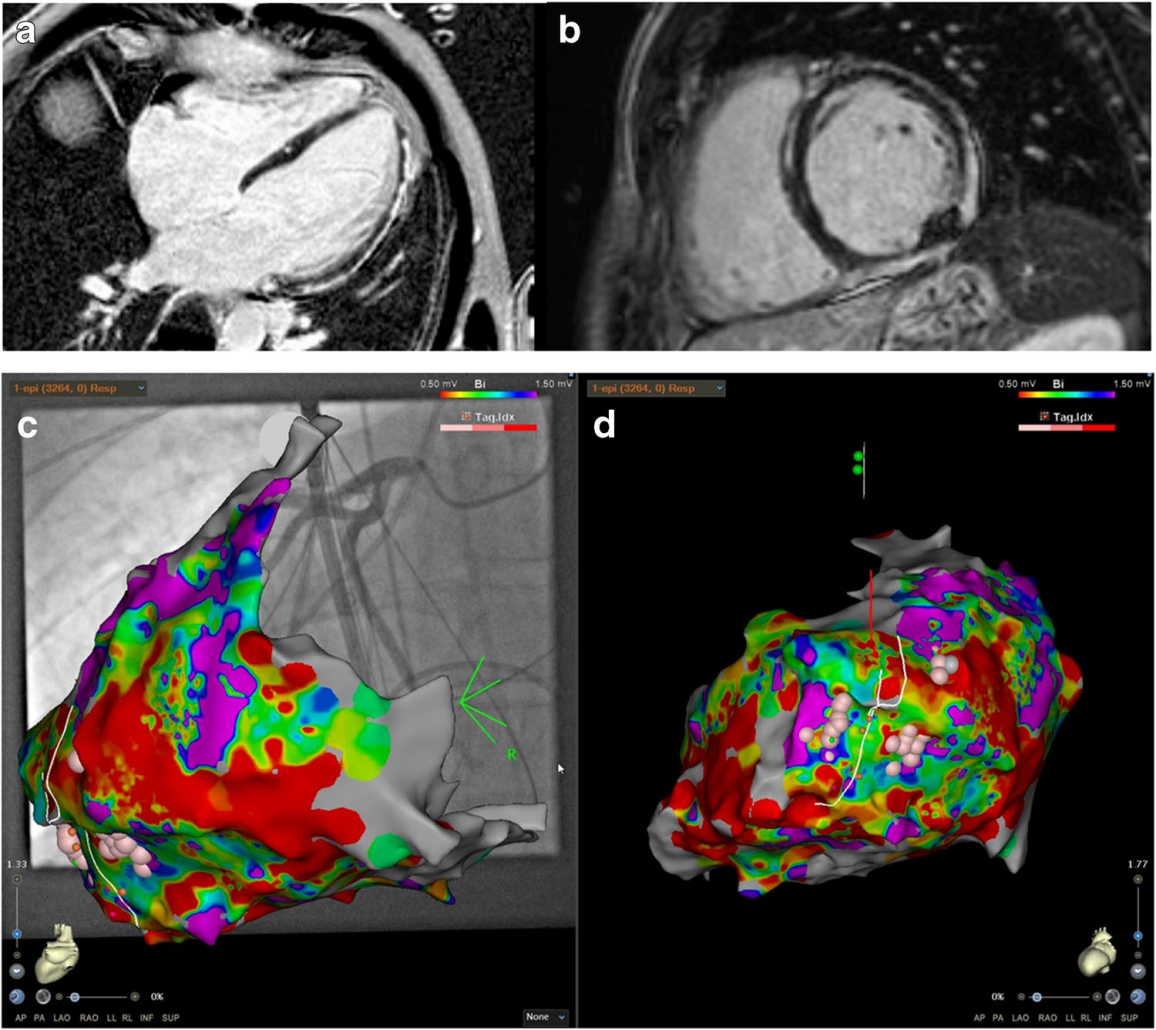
Preprocedural imaging using LGE-CMR to identify epicardial/midmyocardial substrate (A + B). Correspondingly, epicardial (C + D) map. Example of a patient undergoing preprocedural LGE-MRI imaging with evidence of epicardial substrate (A + B) with corresponding VT substrate on the epicardial mapping (C + D) reconstructed with CARTO-3 system (C: PA view showing patchy substrate epicardially, D: left lateral inferior view showing proposed VT channel based on preprocedural CT imaging and respective ablation lesions). The preprocedural MRI showed epicardial and partly intramural substrate inferior and inferolateral as well as inferoseptal
